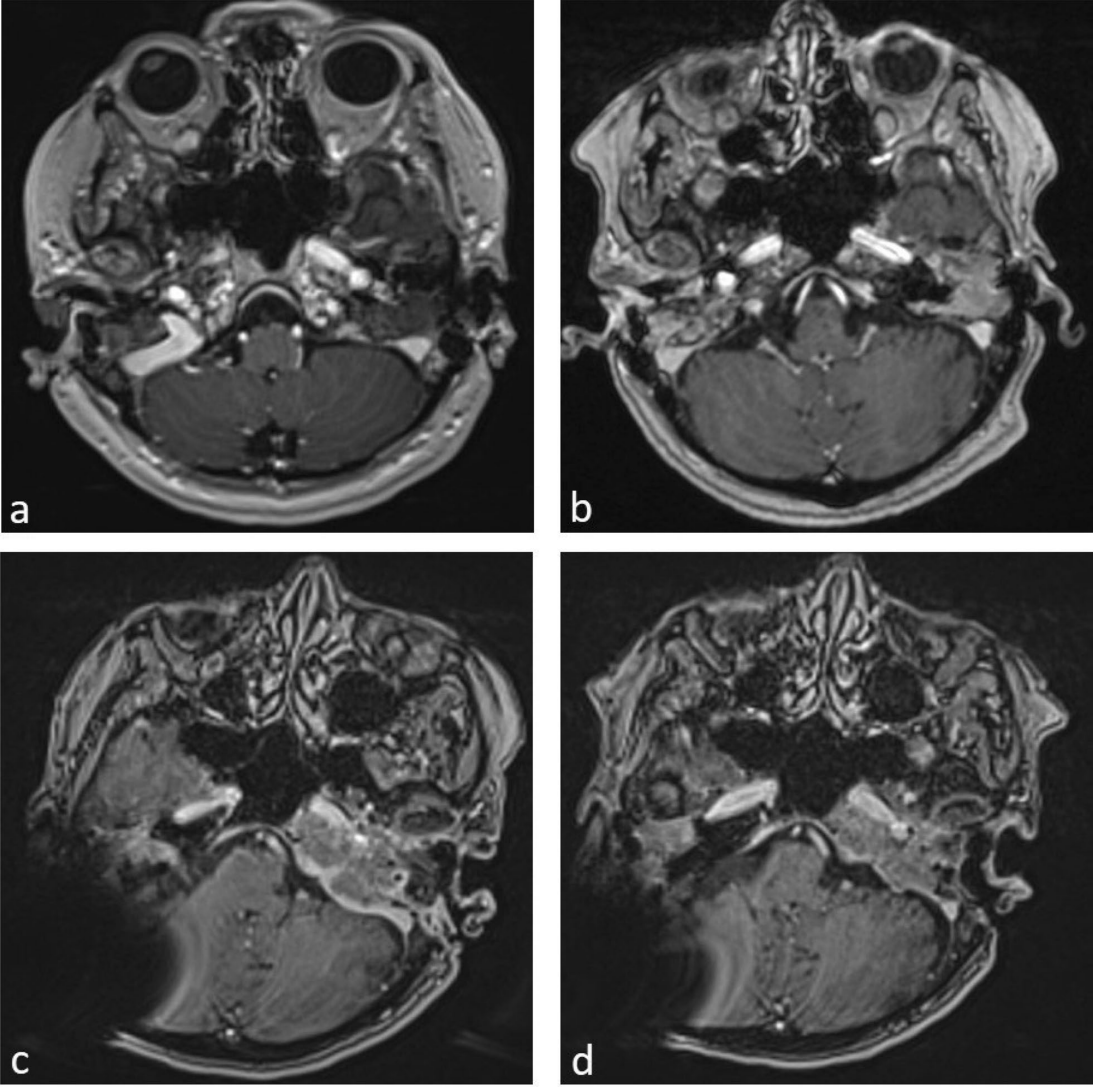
MRI imaging of the SBO’s progression at different points in time (MM/YYYY): a 04/2015: Initial findings. b 08/2019: Progressive bilateral destruction causing sensorineural hearing impairment. c 05/2022: Progress enclosing the left IAC, occlusion of the sigmoid sinus and spreading to the foramen magnum. *(The destruction of the right petrous bone can’t be distinguished due to the MRI signal loss after CI surgery.).*
